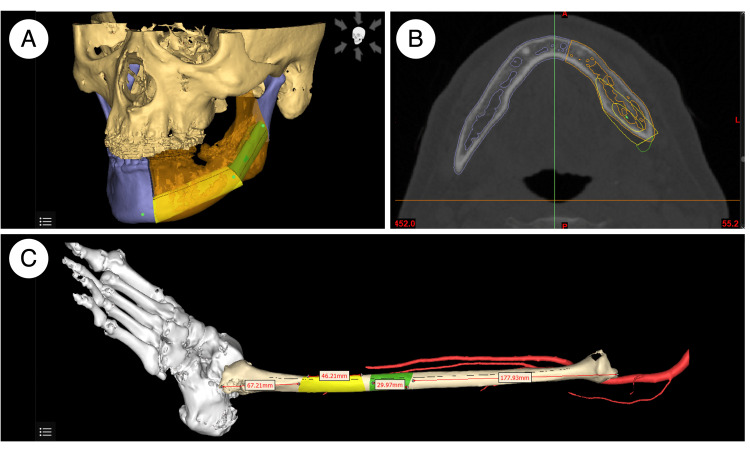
Reconstruction planning using dedicated 3D planning software A: Fibular flap design (3D model); B: Fibular flap design (CT horizontal plane); C: Fibular design
